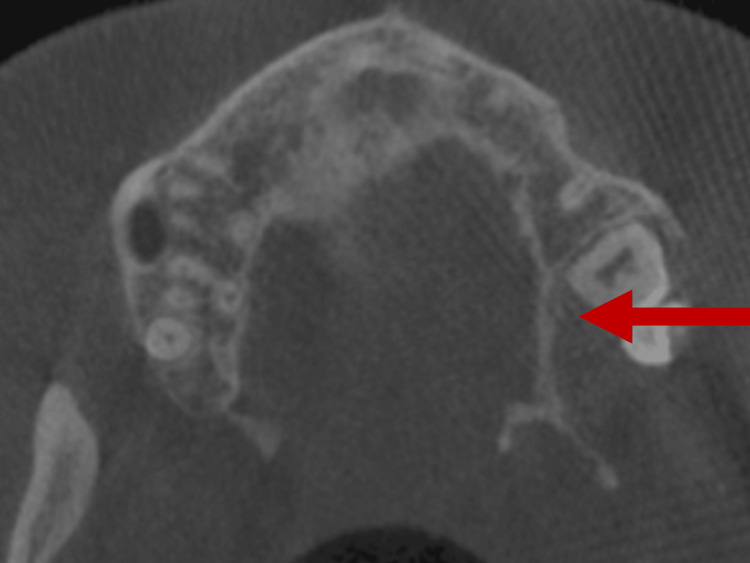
Arrow in cone-beam computed tomography showing the tumor extending into the maxillary sinus and causing bone destruction.
